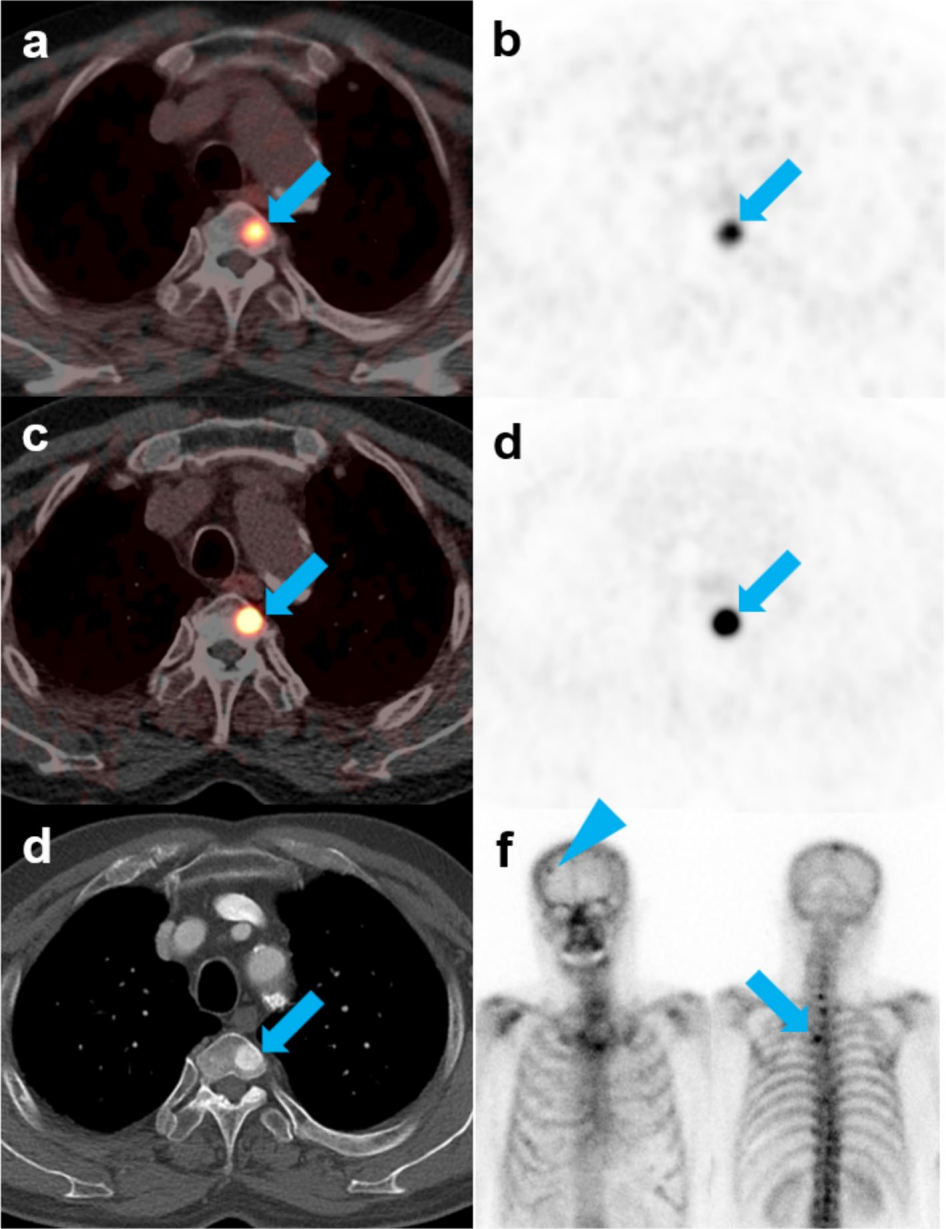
Detection of bone metastases by analog and digital [^68^Ga]Ga-PSMA-11 PET/CT in a patient with prostate cancer who experienced BCR after prostatectomy. A 73-year-old man with prostate cancer was assessed by analog and digital [^68^Ga]Ga-PSMA-11 PET/CT after prostatectomy due to increased serum PSA level (1.12 ng/mL). (a,b) Analog PET/CT images showing a T4 body bone metastasis with SUVmax of 32.5 (arrows). (c,d) Digital PET/CT images showing a T4 body bone metastasis with an SUVmax of 45.2 (arrows). (e) Contrast-enhanced CT showing a T4 body bone metastasis with osteosclerotic changes (arrow). (f) Follow-up bone scan showing bone metastases with increased uptake in the T4 (arrow) and right frontal bone of the skull (arrowhead).
